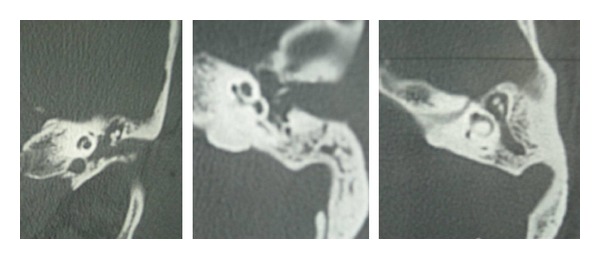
CT in axial and coronal, showing filling of the left middle ear without erosion of its bony walls or ossicles lesions.
